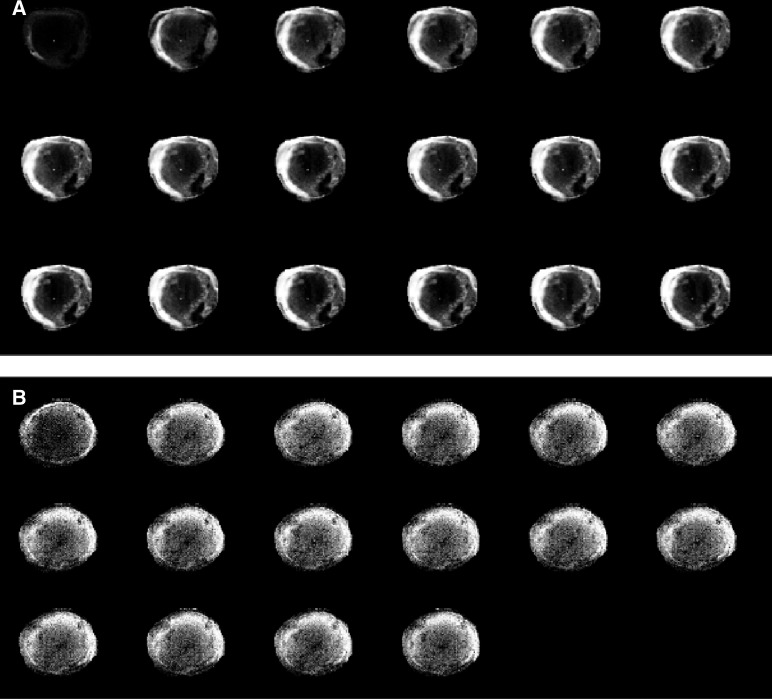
A series of *T*_1_-weighted MR images acquired from a regressive E.G7-OVA tumour (A) and an EL-4 tumour (B) following i.v. injection of the MRI contrast agent, Gd-DTPA. The first images in the series were acquired prior to contrast agent injection. The subsequent images (reading from left-to-right and top-to-bottom) were acquired at 2 min intervals. The presence of the contrast agent increases signal intensity in the images and these increases are proportional to its concentration.
